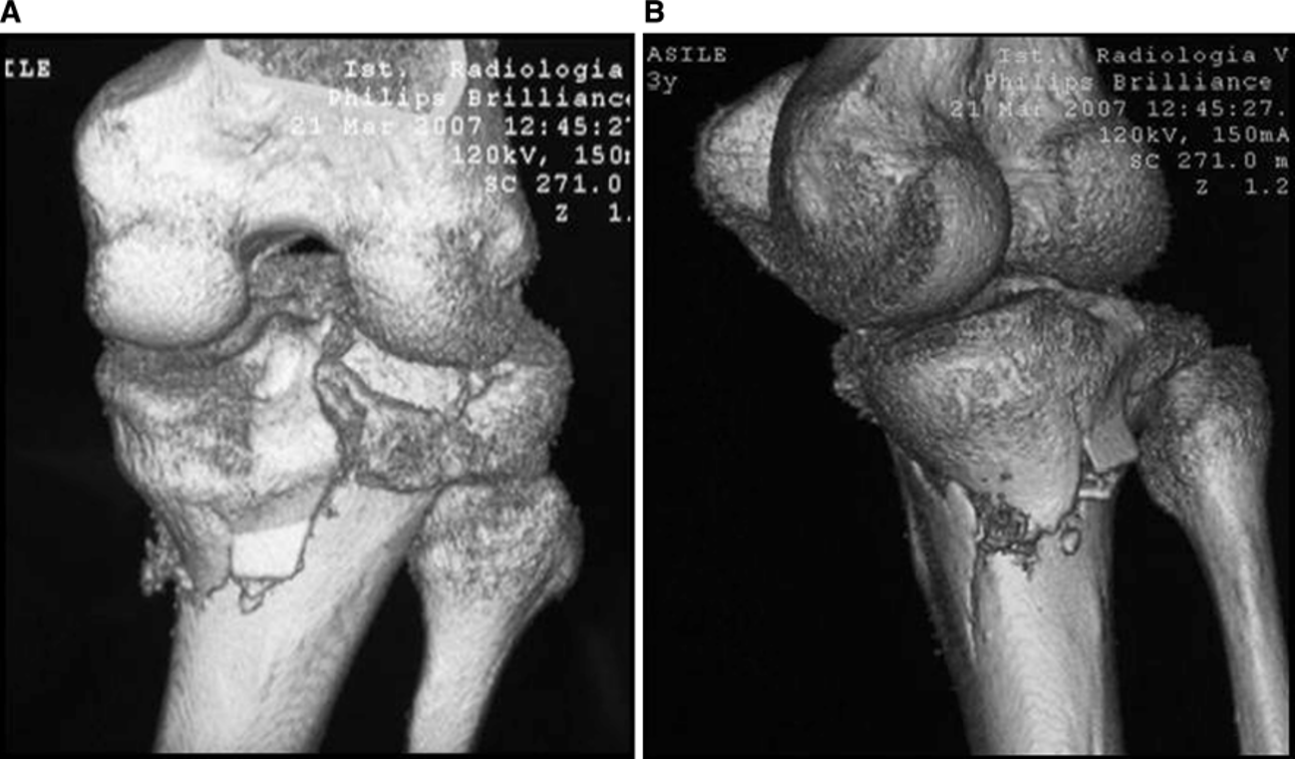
a Schatzker type IV fracture, CT 3D reconstruction. b Schatzker type IV fracture, CT 3D reconstruction
